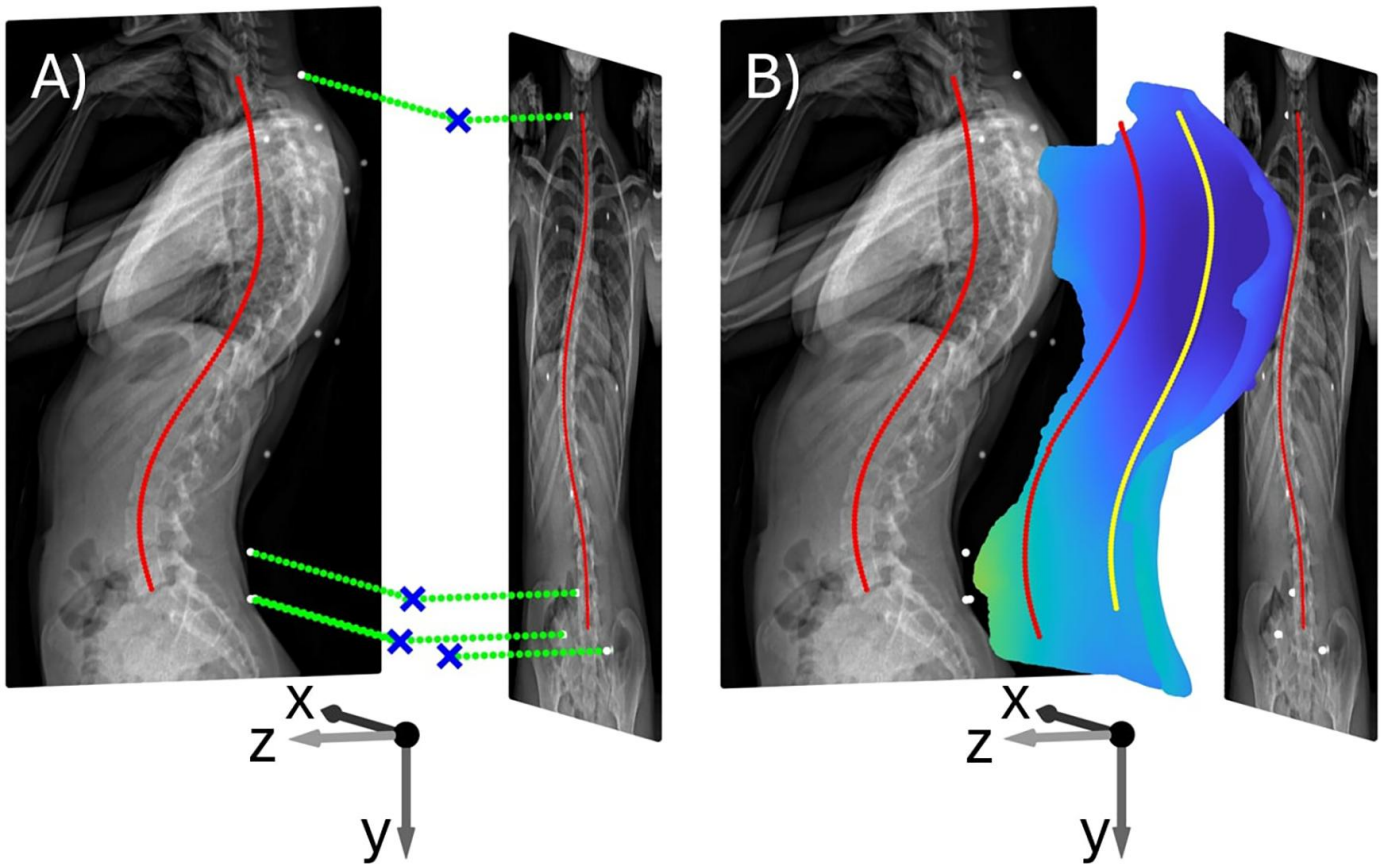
(A) biplanar radiographs with 3D marker positions (blue) from projections (green) of the 2D radiographic marker selections (white). (B) Biplanar radiographs with 3D back shape point cloud (blue), SPL (yellow) and ISL (red) after transformation into the EOS coordinate system (x,y,z).
